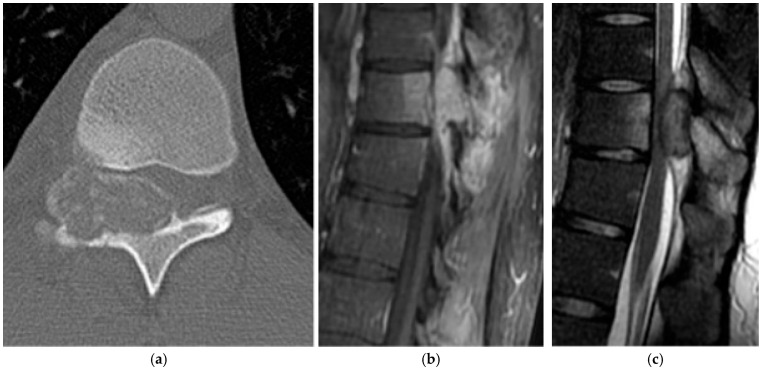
Osteoblastoma. (a) Axial CT bone window, expansile lesion extending from the posterior elements of the T11 vertebral body. The cortex is thin but intact. (b) Sagittal T1 fat saturation post-contrast MR, avid enhancement in the lesion and surrounding marrow and soft tissue representing perilesional inflammation with associated edema. (c) Sagittal T2 MR: the lesion is epidural; the dura is displaced ventrally; perilesional edema is again noted.
